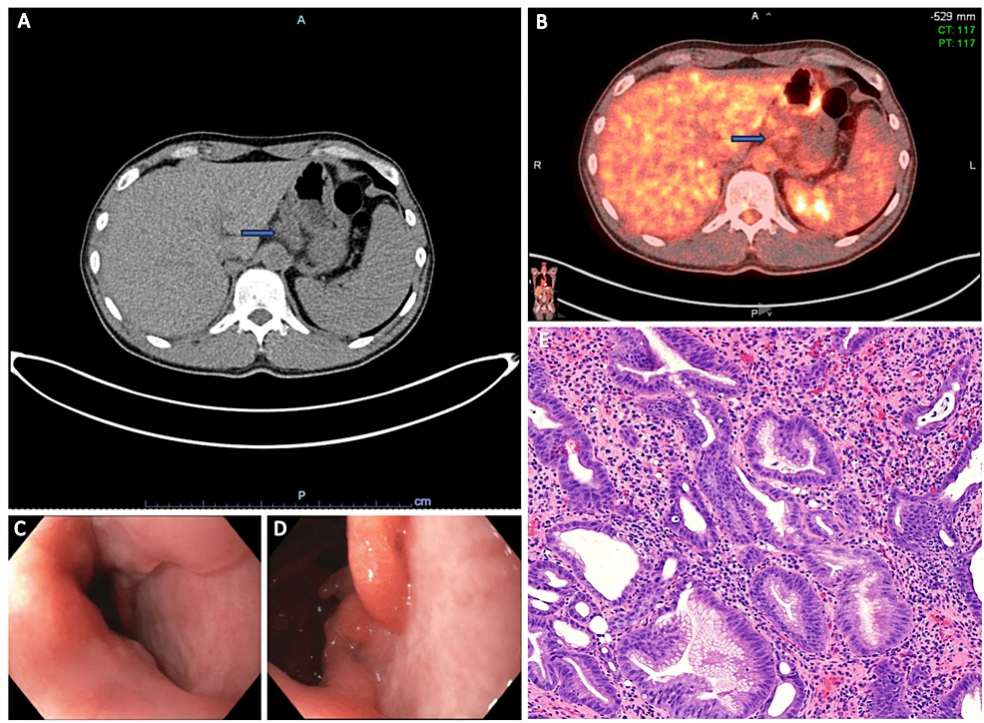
Restaging studies eight months after the start of therapy. (A and B) PET/CT scan with IV F-18 FDG showed heterogeneous soft tissue infiltration along the gastro-hepatic ligament with persistent nodularity measuring up to 2.3 × 2.6 cm, with corresponding mild FDG uptake (SUV max: 2.7) (arrow). (C and D) Upper endoscopy showed an ulcer with elevated borders in the GEJ involving less than one-fourth of the lumen circumference, with no bleeding upon contact. (E) Biopsy of the ulcer at the GEJ showed gastric mucosa with regenerative changes and acute and chronic inflammation of the lamina propria; no residual atypical proliferation of cells was present. PET: positron emission tomography, CT: computed tomography, FDG: fluorodeoxyglucose, SUV max: maximum standard unit value, GEJ: gastroesophageal junction
